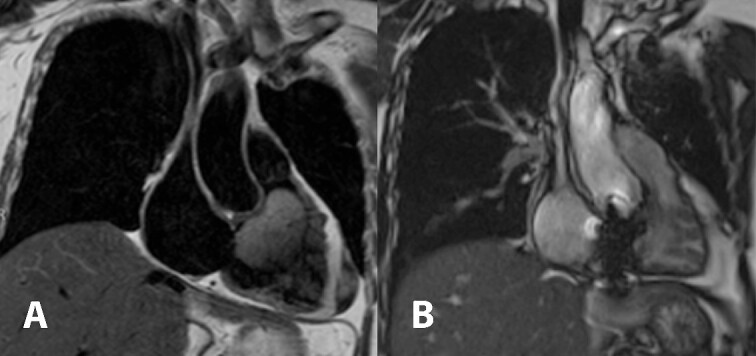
Cardiac magnetic resonance imaging demonstrated T2 hyperintense partially mobile 60 × 39 × 46 mm mass occupying the right ventricular cavity and causing outflow tract obstruction (A). Comparison to post-surgical resection in picture (B) showed decreased right atrial and ventricular size, the tricuspid bioprosthetic valve in place, and right ventricular function returned to normal.
